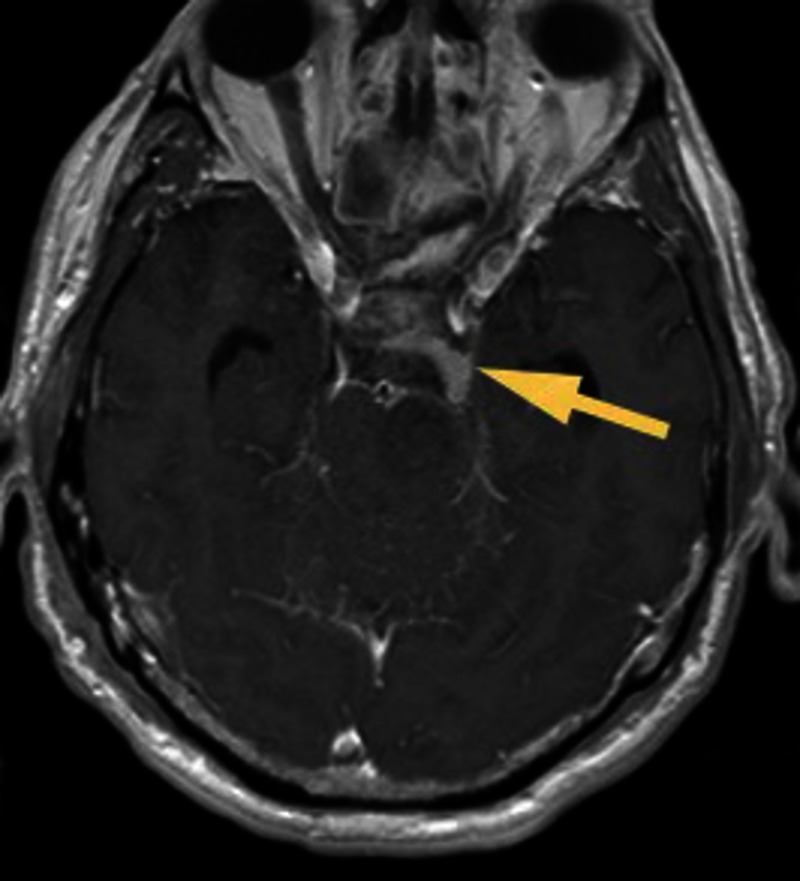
Postoperative MR Imaging Postoperative axial MRI with contrast revealing the degree of resection. The pons is completely decompressed. The arrow is depicting a small tumor residual posterior to the cavernous sinus region.
